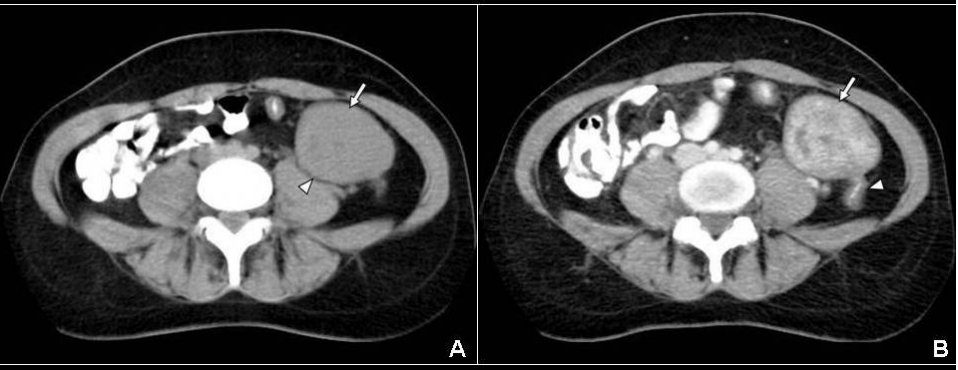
A, B. A: Axial non-enhanced CT scan at the level of aortic bifurcation, demonstrates a rounded, well-circumscribed, mass of soft tissue density (arrow) in the left lower quadrant abdomen. The mass abuts the left psoas muscle (arrowhead) showing an intact interface with it. B: Contrast-enhanced CT scan at the same level with figure 3A, exhibits marked heterogeneous enhancement of the mass (arrow). Note the contrast-filled bowel loop being in direct proximity with the posterior surface of the mass (arrowhead).
